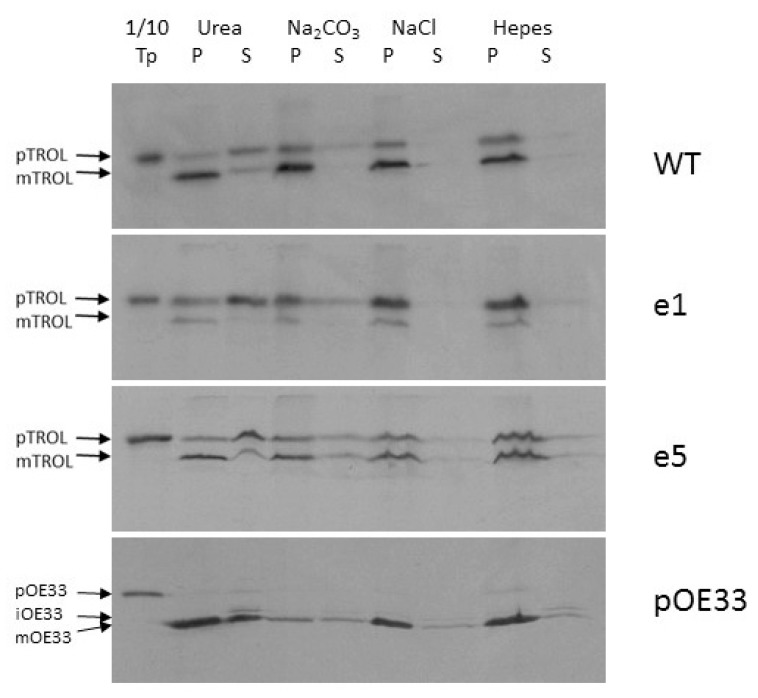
Extraction of wt TROL and its presequence mutants e1 and e5 from the membranes. In vitro synthesized [^35^S]-TROL, e1, e5, and control protein pOE33 were incubated with isolated intact chloroplasts at 25 °C for 20 min, in a standard import reaction containing 3 mM ATP. Subsequent to import, chloroplasts were re-isolated, washed, and separated into membrane and soluble fractions. Isolated membranes, containing imported proteins, were treated with 6 M Urea in 10 mM HEPES/KOH pH 7.6 (lanes 2 and 3), 0.1 M Na_2_CO_3_ pH 11.5 (lanes 4 and 5), or 1 M NaCl (lanes 6 and 7). All incubations were performed for 20 min on RT. As a control, membranes were incubated solely in 10 mM HEPES/KOH pH 7.6 for 30 min on ice (lanes 8 and 9). Afterwards, samples were centrifuged at 265,000× *g* for 10 min at 4 °C, and both pellets (P, lanes 2, 4, 6, and 8) and the supernatants (S, lanes 3, 5, 7, and 9) were analyzed by SDS–PAGE and by exposure on X-ray films. Lane 1 represents 10% of the respective translation product (Tp) used for the import reactions. The positions of pTROL, mTROL, pOE33, iOE33, and mOE33 are indicated by arrows.
